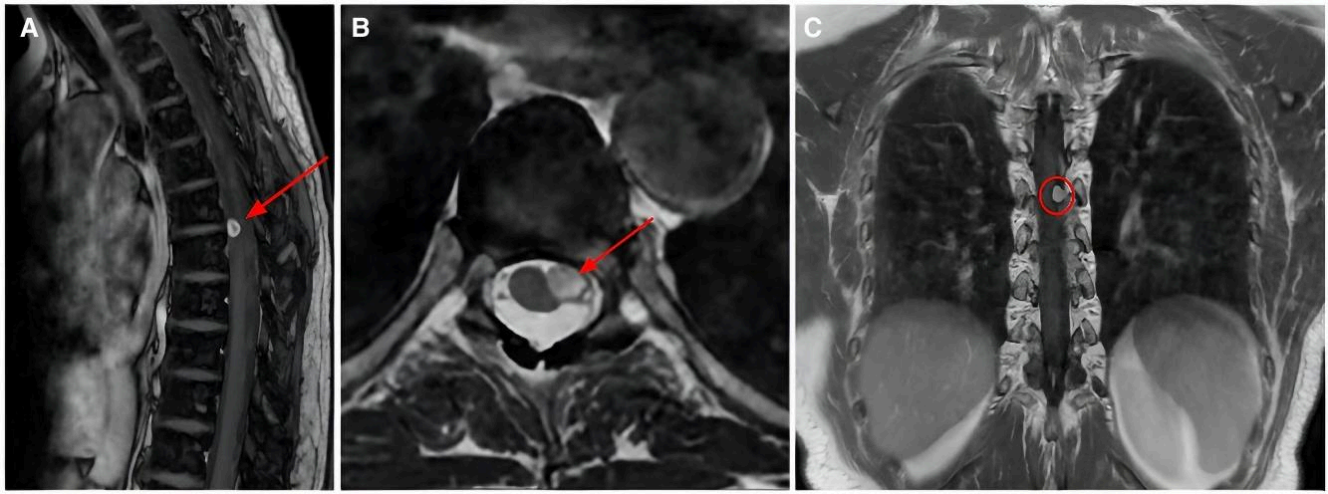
Magnetic resonance imaging of the thoracic spine. Axial (*A* with an arrow), sagittal (*B* with an arrow), and coronal (*C* within the circle) T2-weighted images reveal the presence of a left-lateral D6–D7 extramedullary intradural expansive lesion, consistent with a schwannoma. MRI, magnetic resonance imaging.
